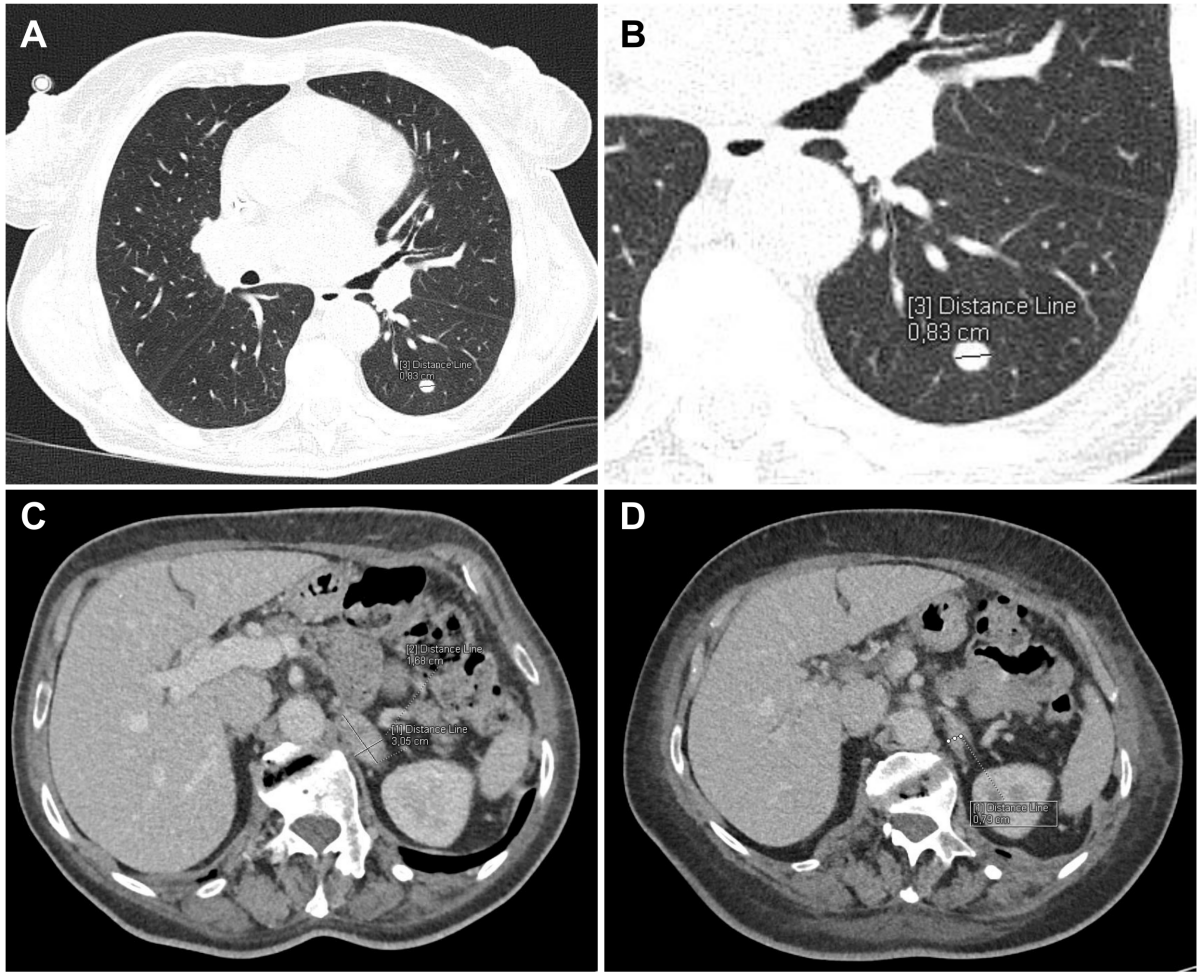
Contrast-enhanced CT of the thorax, abdomen, and pelvis. (A, B) CT scans of the lungs showing a well-circumscribed metastatic nodule (8 mm) in segment 6 of the left lung. (C) CT scan of the abdomen (venous phase) demonstrating a metastatic mass (30 × 17 mm) in the left adrenal gland. (D) CT scan of the abdomen performed 3 months earlier (venous phase) showing the left adrenal gland without metastatic changes.
